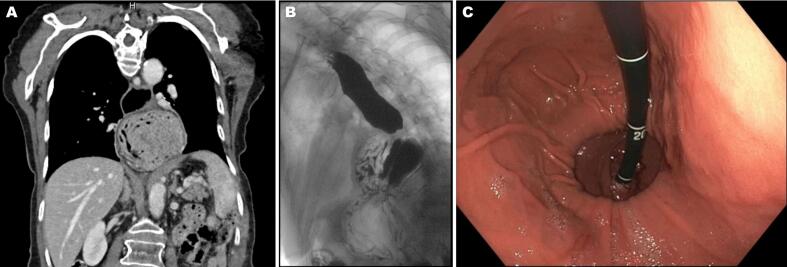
Preoperative thoracoabdominal computerized tomography (CT), barium esophagram, and endoscopic images: A) Coronal view of a contrast-enhanced CT slice demonstrates the paraesophageal hernia with a large portion of the stomach within the thoracic cavity. B) Barium swallow study shows large paraesophageal hernia with poor clearance of barium and dilated esophagus. C) Retroflex view of the gastroesophageal junction shows a 5 cm large paraesophageal hernia with a sliding component.
